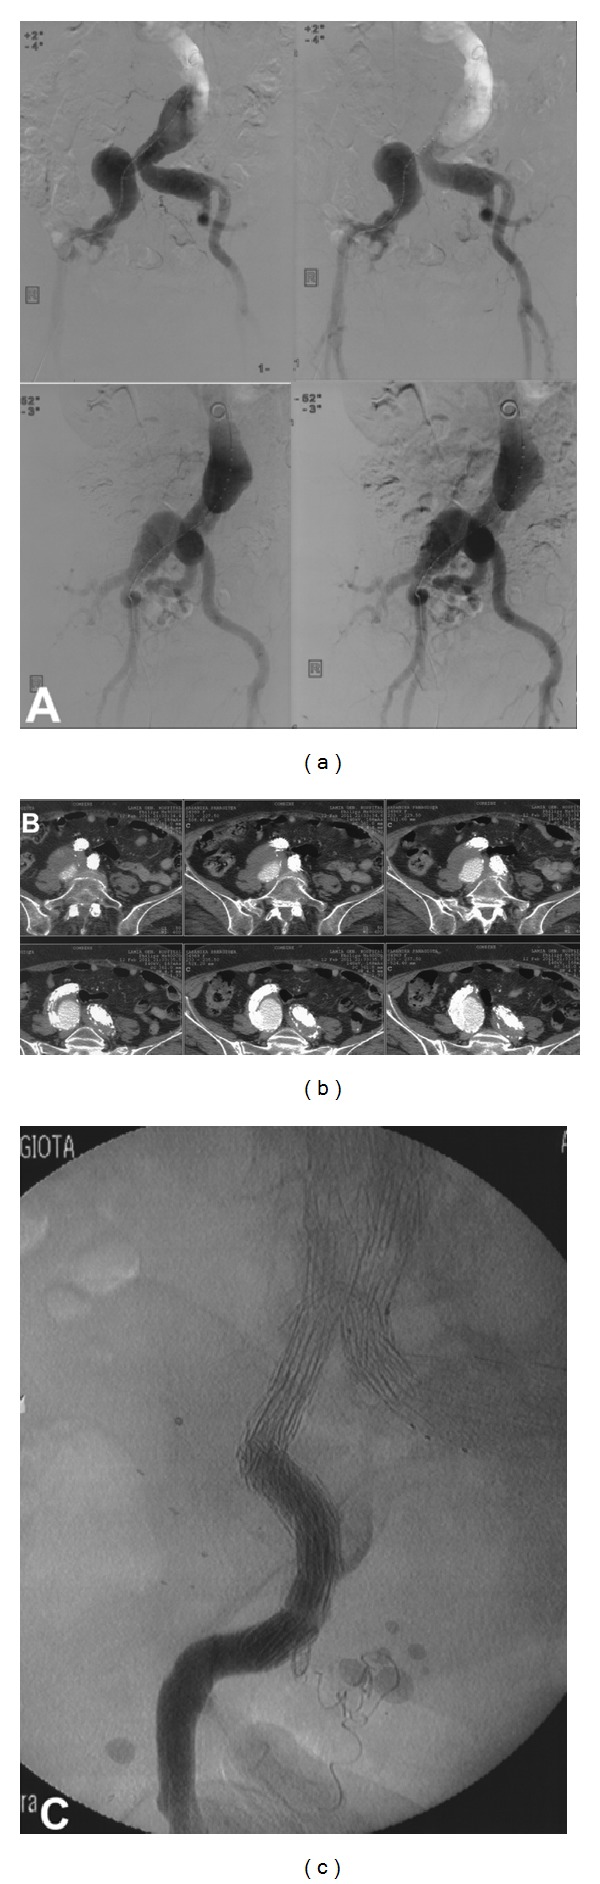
(a) A complex aortoiliac aneurismal pattern with bilateral CIAA and AAA. (b) Follow-up CT scan in one year showing endoleak type Ib in the flared right CIA and aneurysm sac expansion. (c) Completion angiography after right IIA embolization and extension stent grafts from right CIA to right EIA.
